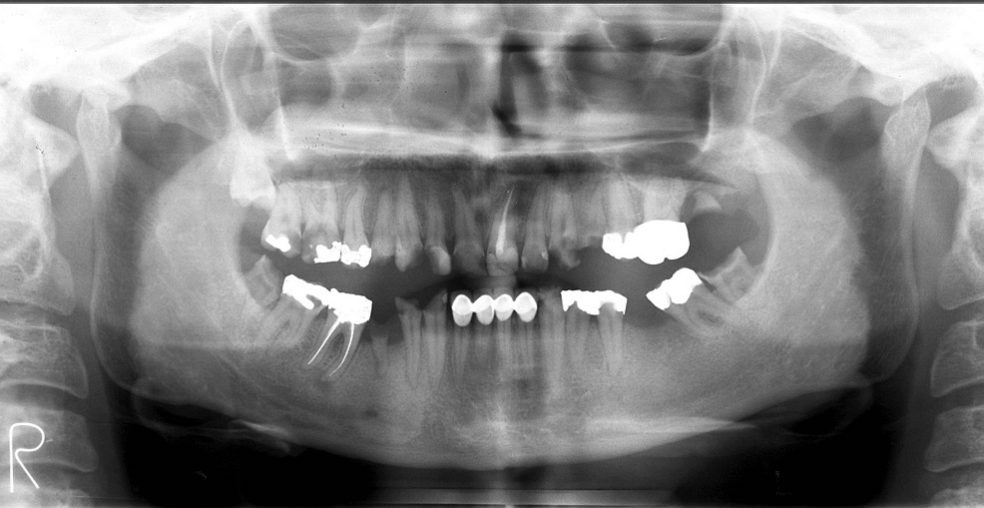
Pre-treatment - panoramic X-ray
